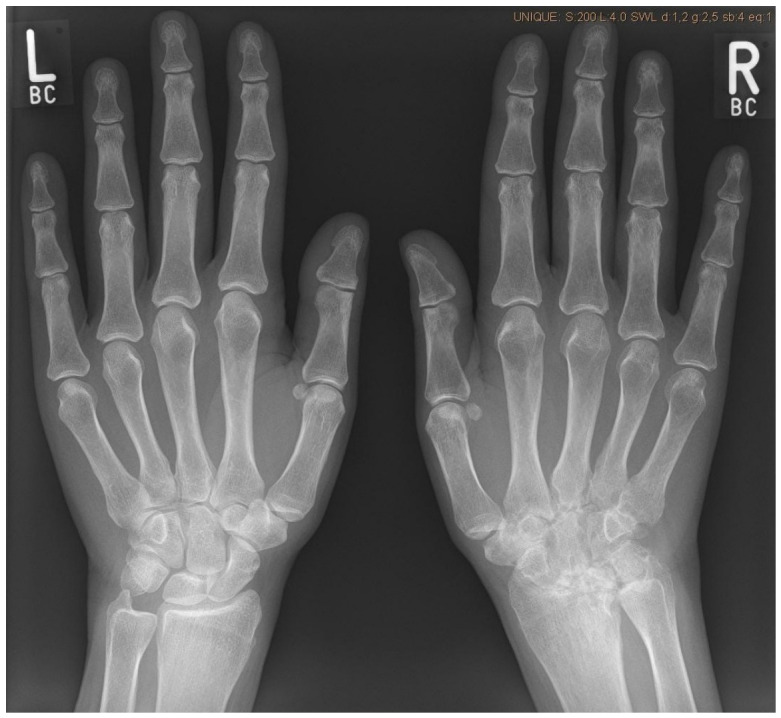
X-ray of both hands. Different stages of destruction of the carpal row between the right and left sides. © C. Biehl.
